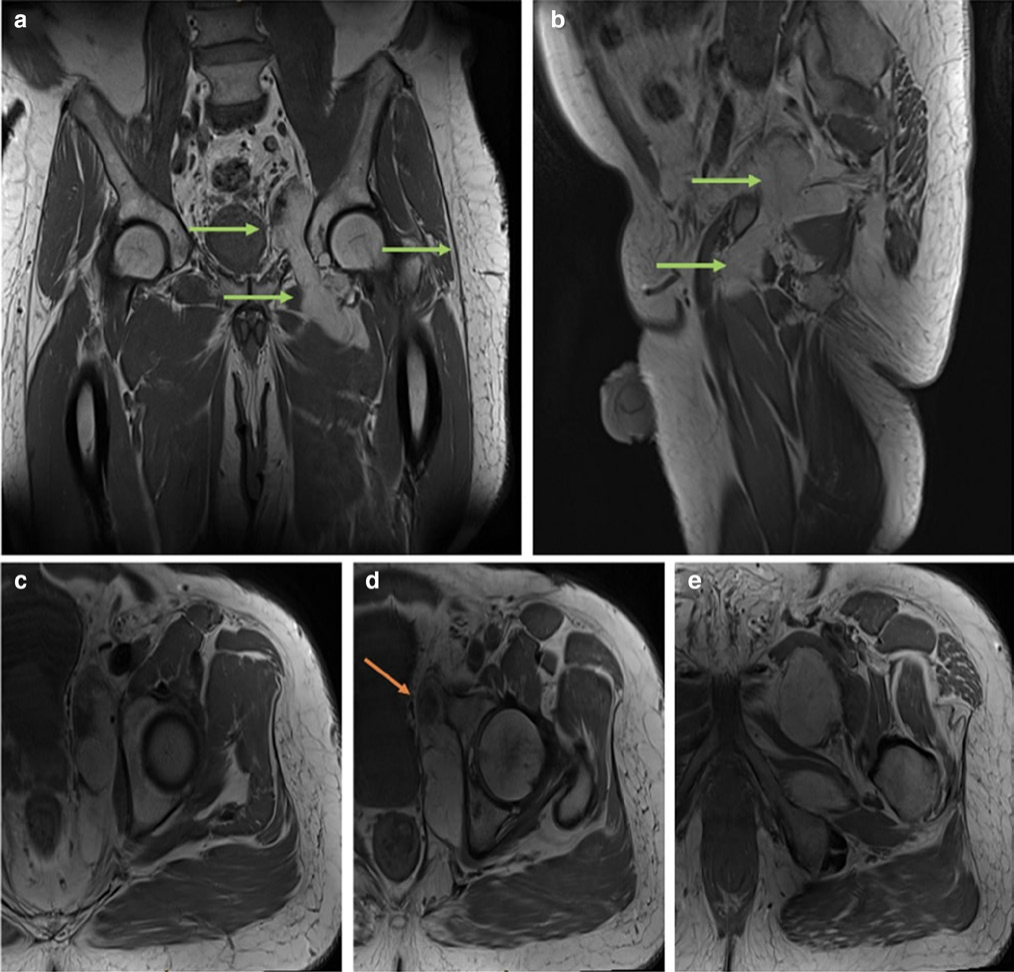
(a – e) Magnetic resonance imaging (MRI): Corresponding coronal, sagittal and axial *T_1_*-weighted sequences demonstrating bright fatty signal within the lesion (note of same signal with the subcutaneous fat). Green arrows demonstrating normal fat. Orange arrows demonstrating abnormal aggressive features. 3d. Solid nodule (low signal compared to the fat) within the lesion.
